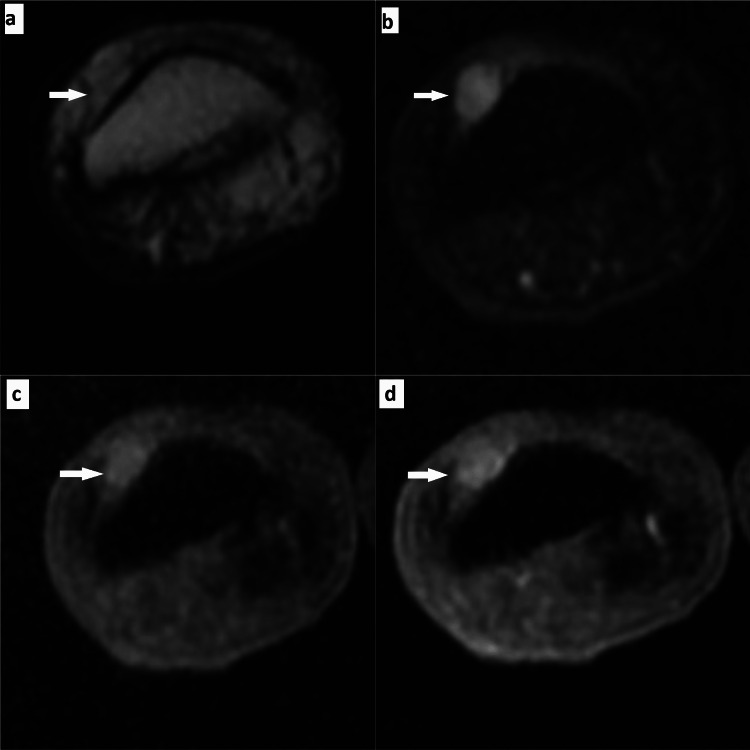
MRI findings of a great toe lesion Axial T2-weighted image shows an iso-intense lesion in the subungual aspect of the great toe (a). Short inversion time inversion recovery (STIR) images suggest the lesion was more conspicuous (b) Hypo-intense on a T1-weighted, fat-saturated image (c). Minimal to mild post-contrast enhancement was seen on T1 post-gadolinium fat saturation images (d).
